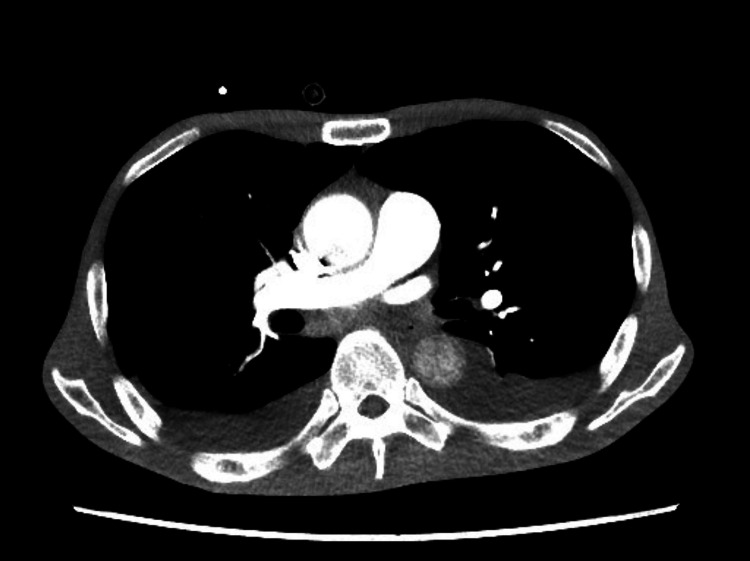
Contrast-enhanced computed tomography of the chest demonstrates bilateral pleural effusion but no pulmonary emboli.
